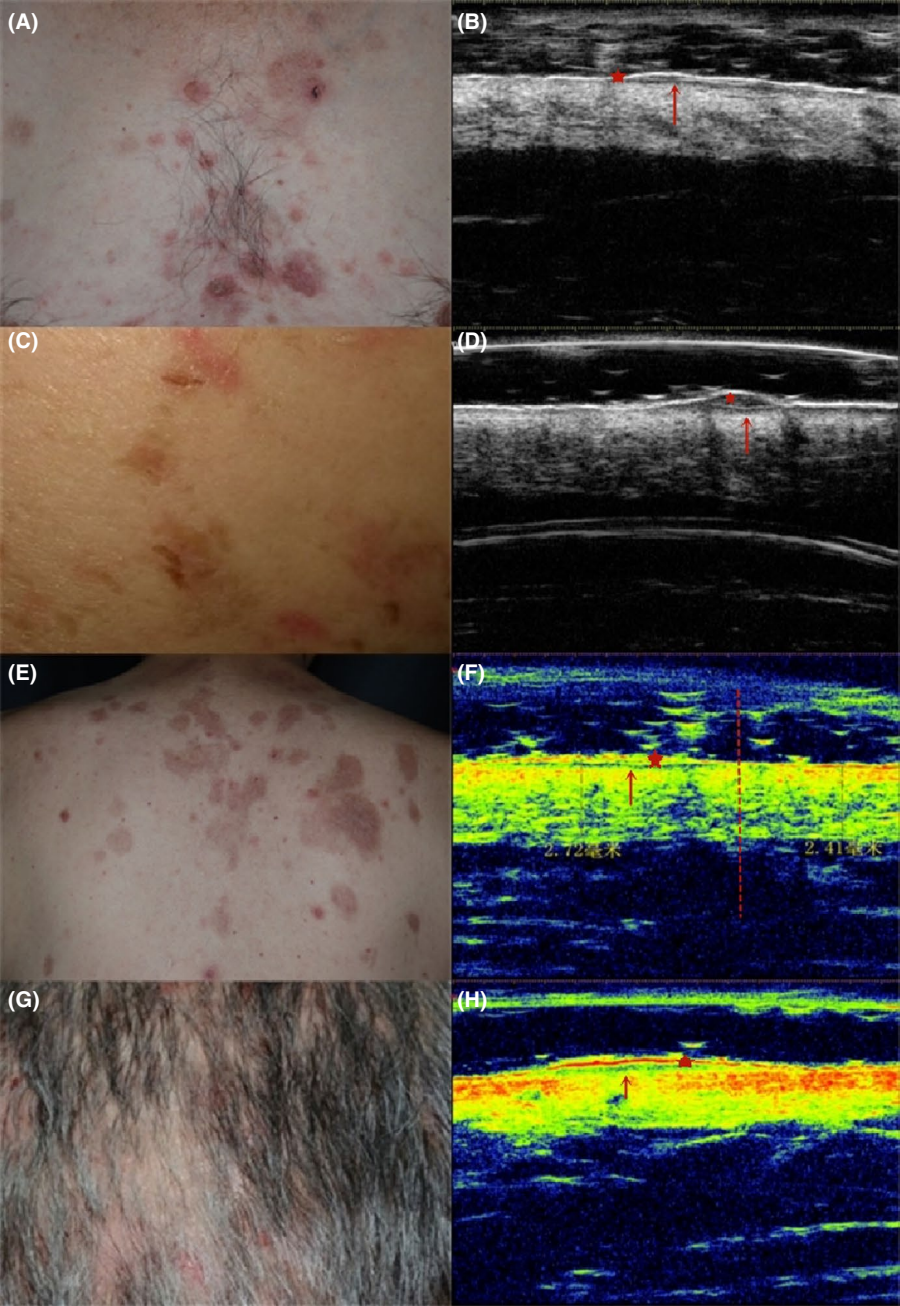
Clinical and ultrasonographic images of early‐stage PV patient. 1A, C, E, G, Macroscopic images. 1B, D, F, H, Corresponding ultrasonographic images. 1B, H, echo enhancement of epidermis and echo reduction of the dermis. 1B, D, F, H, linear or oval intra‐epidermal hypoechoic region (*), linear anechoic band at dermal‐epidermal junction (↑). 1B, 1D, 1F the thickness of the dermis is slightly increased
